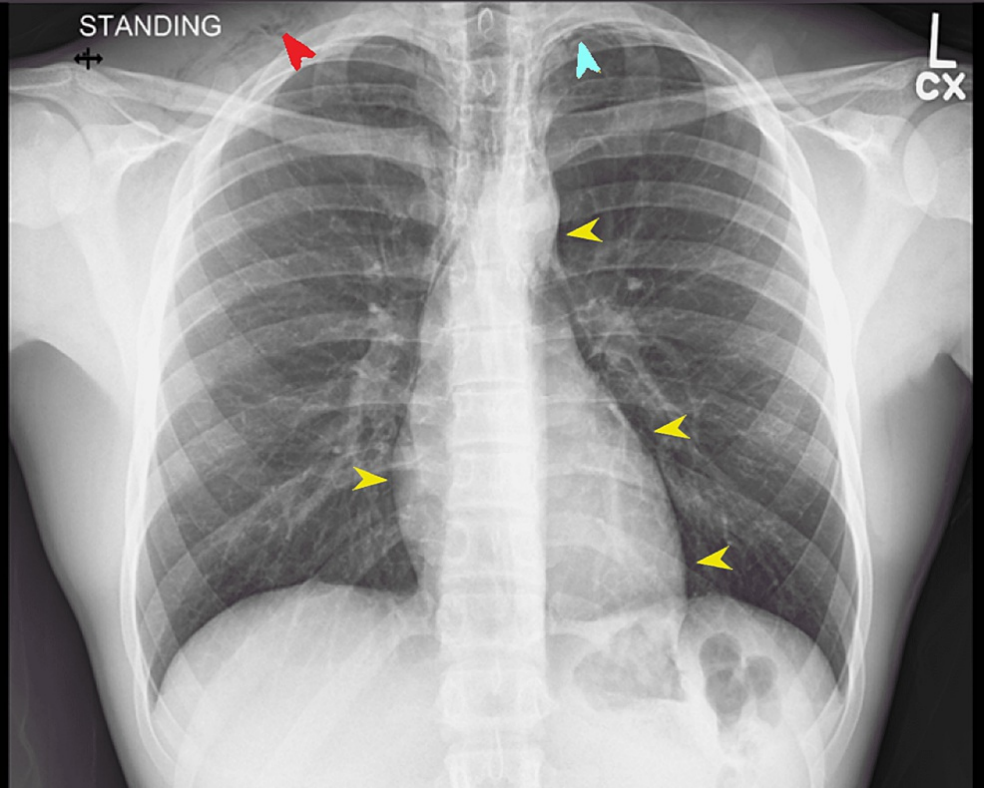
AP chest X-ray Chest X-ray demonstrates pneumomediastinum (yellow arrows), with an apical pneumothorax (blue arrow), and free air in the supraclavicular soft tissue (red arrow)
